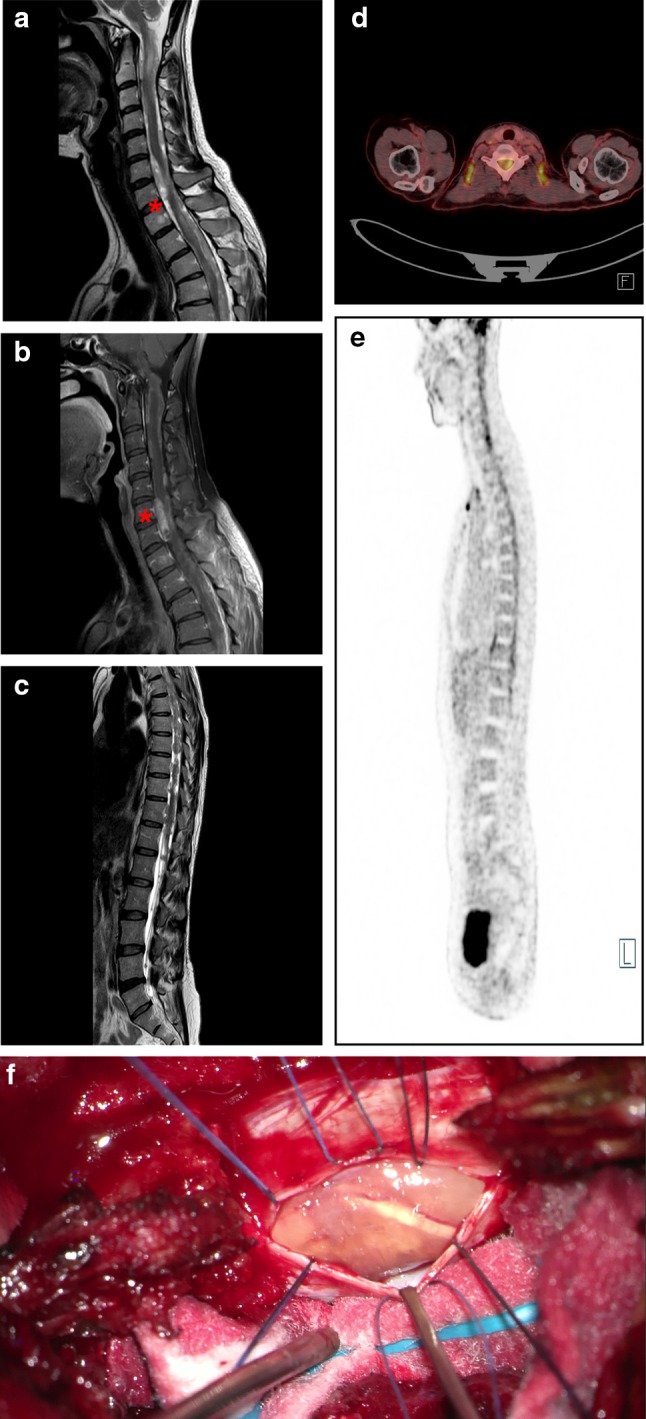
Radiological scans and intraoperative photograph of patient 12 a sagittal cervical MRI-T2 scan, red asterisk marks intradural, extramedullar tumor (C7) b sagittal cervical contrast enhanced MRI-T1 scan c sagittal MRI-T2 scan, the spinal canal is filled with widely disseminated tumor masses d and e F-18-FDG PET-CT (transversal, C7 (d) and sagittal, whole spine (e)) shows raised metabolism corresponding with the tumor at level C7 f intraoperative photograph taken during initial biopsy showing intradural mass
